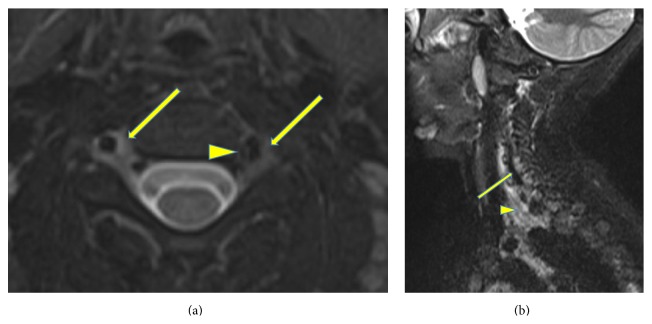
Imaging of cervical area at diagnosis. (a) Baseline MRI T2 weighted images showed moderate adventitial thickening of both the vertebral arteries at the level of C3 (thin yellow arrows) with adjacent soft tissue edema. There were focal areas of irregularity causing moderate stenosis on the left side (arrowhead). (b) Sagittal view of cervical MRI demonstrated poststenotic dilation of his left vertebral artery (arrow) and marked myositis (arrowhead).
